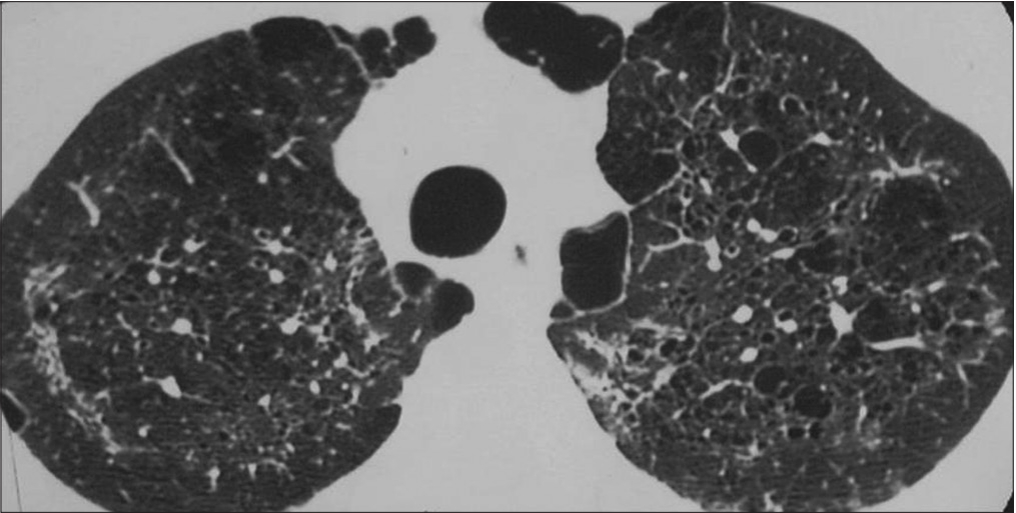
Pneumocystis carinii* pneumonia. Lung cysts are usually multiple and bilateral, but range in size, shape and distribution. They are more commonly appreciated on computed tomography (CT)/high-resolution CT
